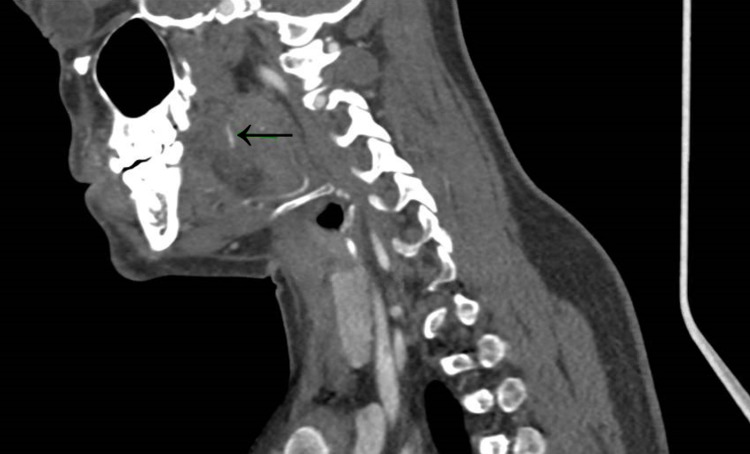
Sagittal contrast-enhanced CT of neck: suspected intraglossal fish bone visible in caudocranial orientation Black arrow points to the suspected fish bone
